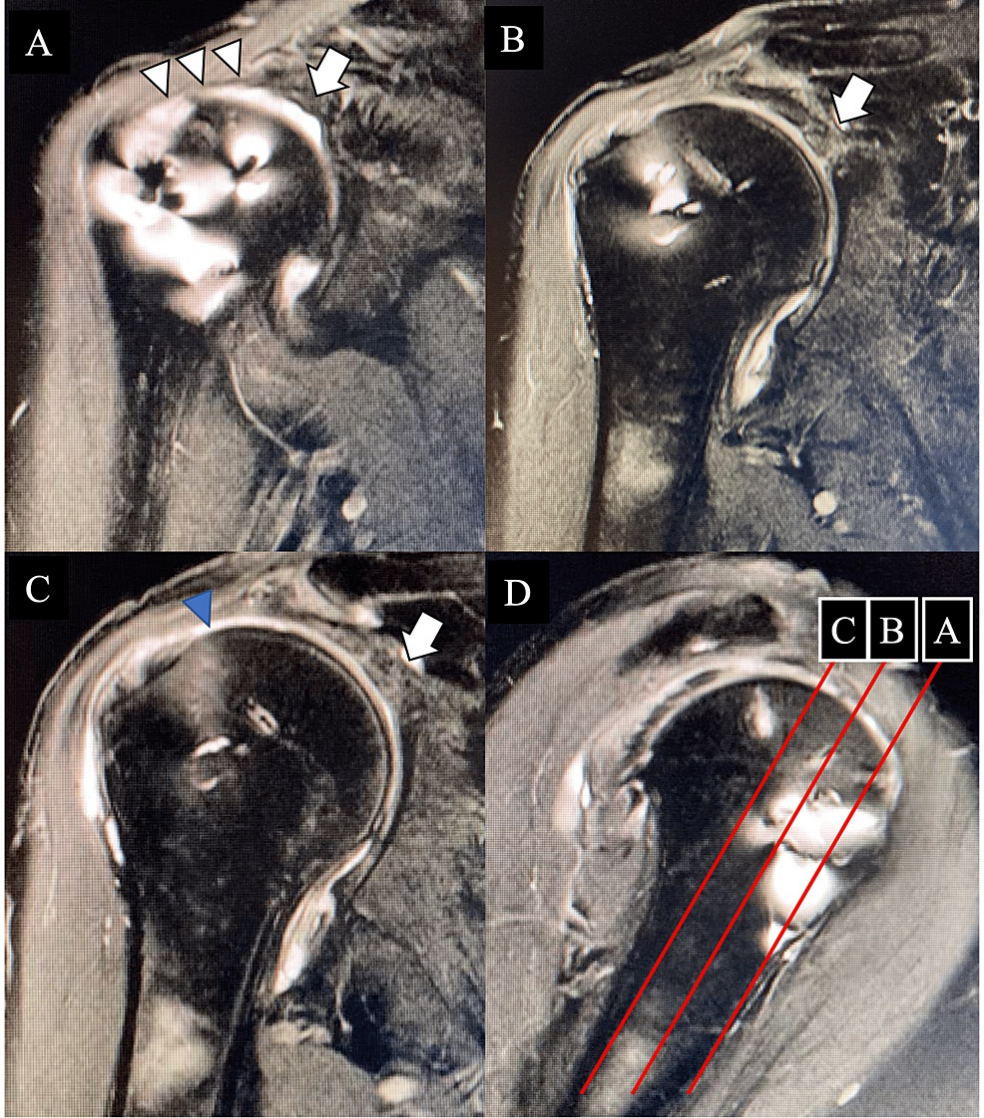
Magnetic resonance imaging of the right shoulder at 21 months after the primary surgery The oblique coronal (A-C) plane shows allograft engraftment was obtained at the glenoid side (white arrow). However, the allograft at the humeral side is considered to be partially ruptured (white triangle, considered engraftment area; blue triangle, ruptured area) (D) Red lines showed each coronal slice.
